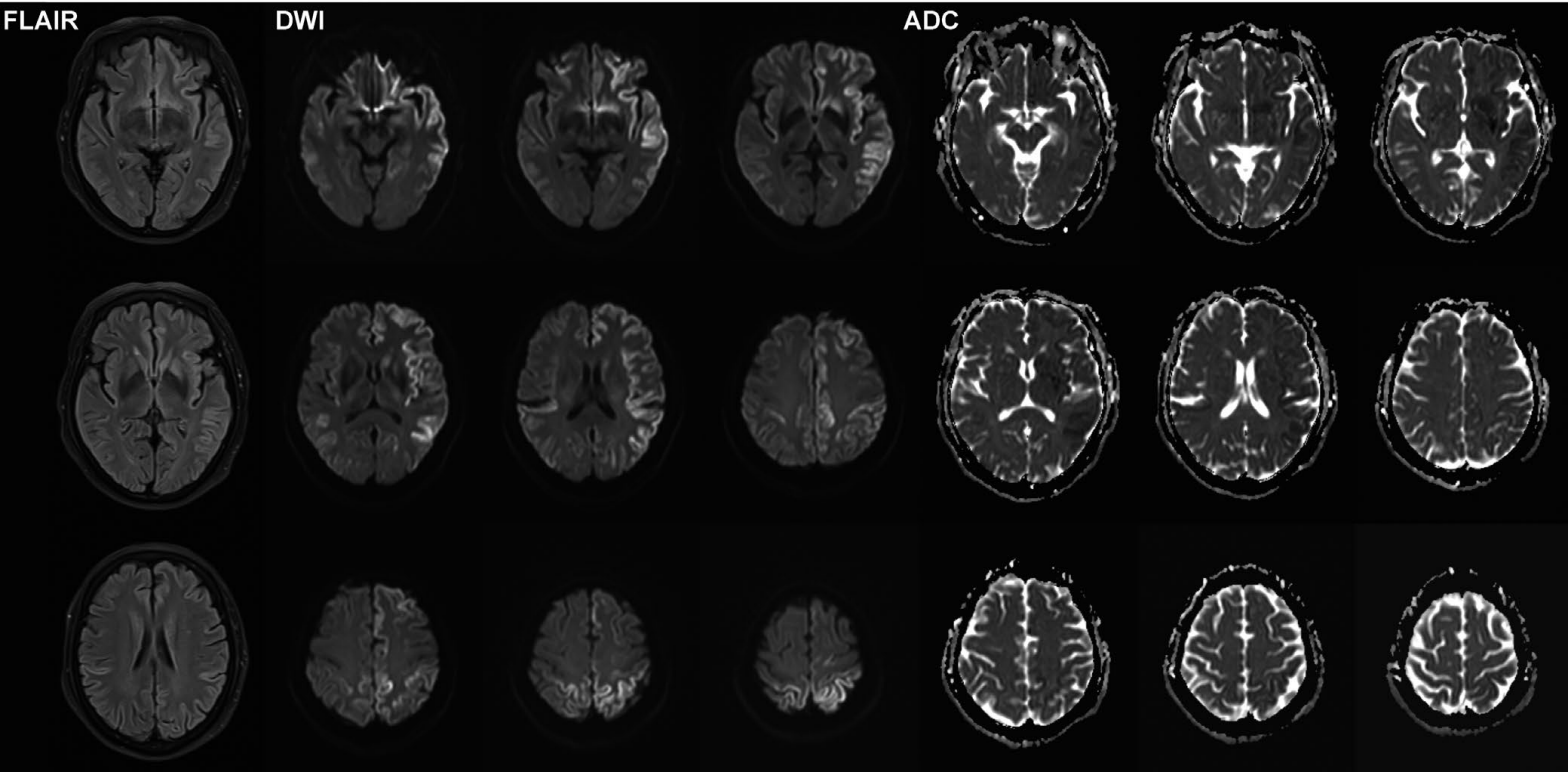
There was an increased signal intensity of the bilateral frontal‐temporal‐parietal cortex in the diffusion‐weighted imaging(DWI) sequence. In contrast, there was a decreased signal intensity of the counterpart cortex in the apparent diffusion coefficient (ADC) sequence. The signal hypointensity on the FLAIR sequence is less evident than the DWI sequence
